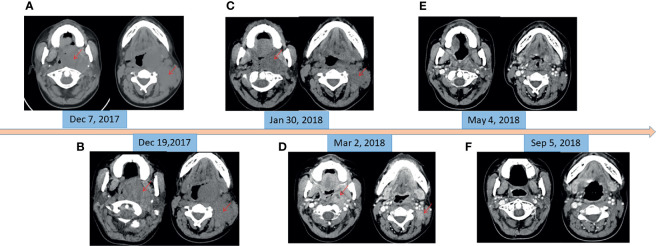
CT imaging of changes of masses in oropharynx and left neck. (A) Before chemotherapy (pharyngeal mass: 6.0 cm × 4.6 cm; neck mass: 3.9 cm × 2.9 cm). (B) At the beginning of radiotherapy and 11 days after chemotherapy (pharyngeal mass: 6.0 cm × 4.4 cm; neck mass: 3.5 cm × 2.8 cm). (C) At the end of the radiotherapy and oral crizotinib for 1 month (pharyngeal mass: 4.3 cm × 2.7 cm; neck mass: 2.6 cm × 1.4 cm). (D) At the beginning of oral imatinib and thalidomide (pharyngeal mass: 2.8 cm × 1.9 cm; neck mass: 2.1 cm × 1.4 cm). (E) Oral imatinib and thalidomide for 2 months. (F) Oral imatinib and thalidomide for 6 months.
